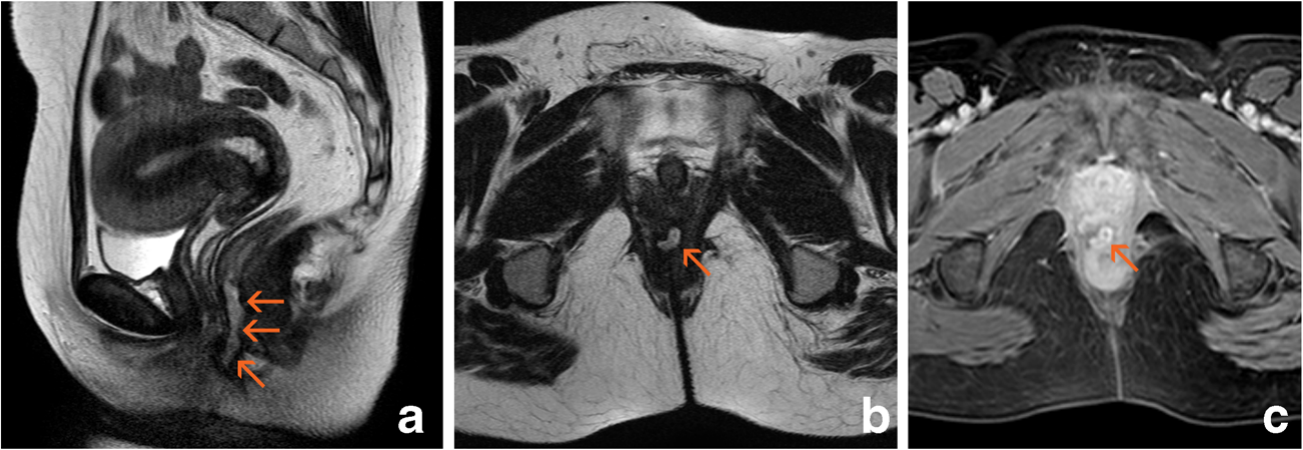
Anovaginal fistula. A thick gauge fistula exibited abscess formation involving the recto vaginal septum (arrows). a Sagittal T2-weighted image. b Oblique-transverse T2-weighted image. c Post-gadolinium oblique-transverse fat-supressed gradient echo T1-weighted image
